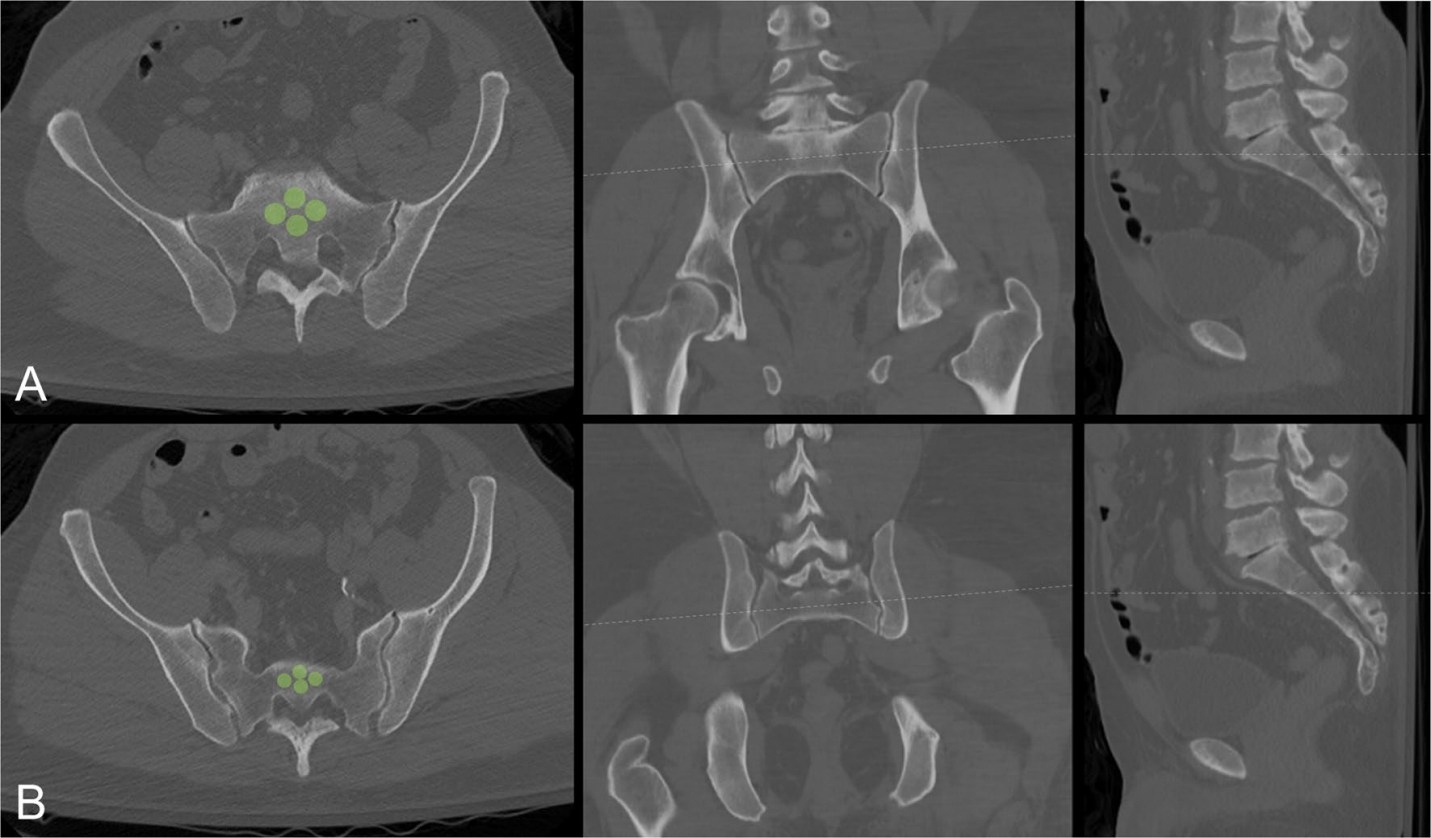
Axial, sagittal, and coronal CT images depicting cross-referencing technique used for localization of regions of interest (ROIs) represented by green circles in (a) S1 and (b) S2
